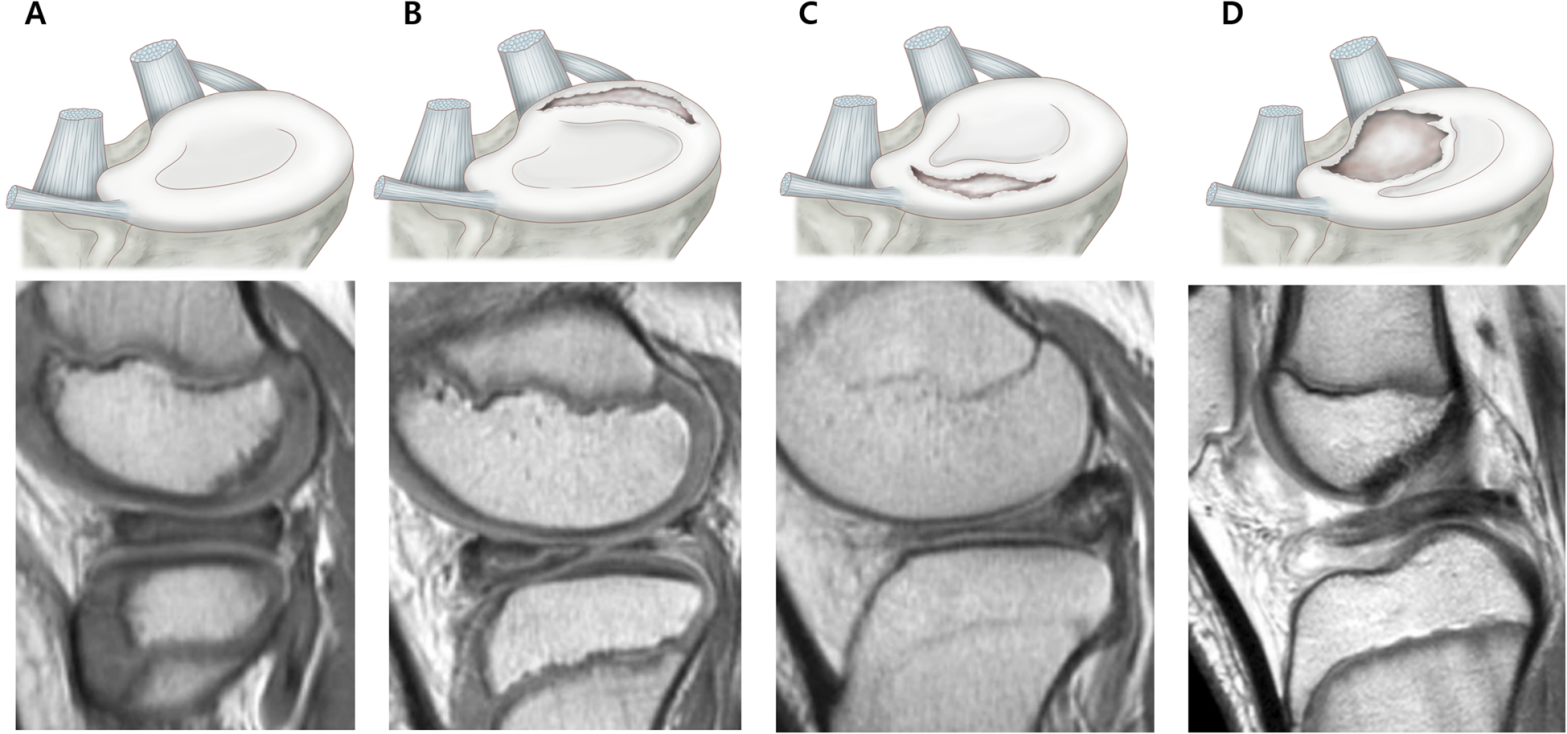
Magnetic resonance imaging classification of the discoid lateral meniscus (DLM) based on peripheral detachment. a No shift, b anterocentral shifting corresponding to a longitudinal tear in the posterior horn, c posterocentral shifting corresponding to a longitudinal tear in the anterior horn, and d central shifting corresponding to posterolateral corner loss
